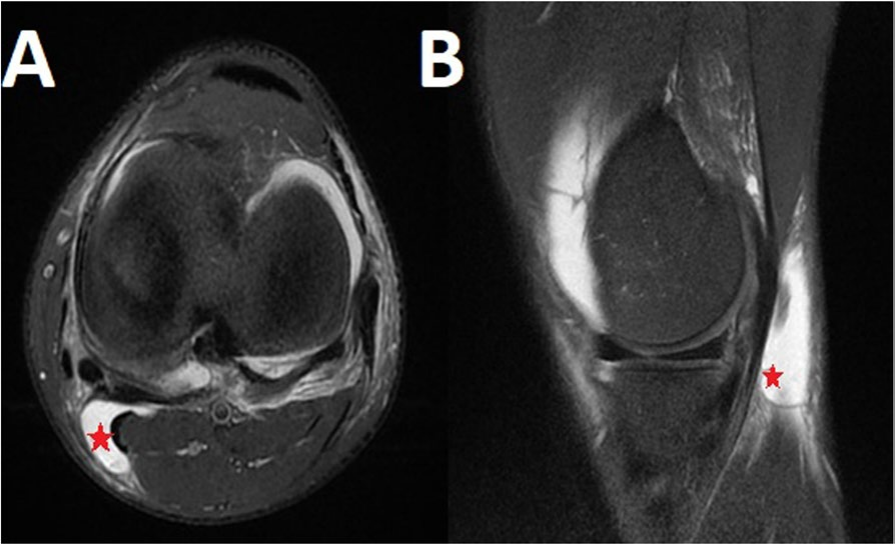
MRI of *case 1*. Left knee axial view (A) and coronal view (B). These MRI slices show the hyperintensity signal indicative of a fluid collection in the posteromedial muscle plane (red star). The direct arm of the semimembranosus tendon appears intact
